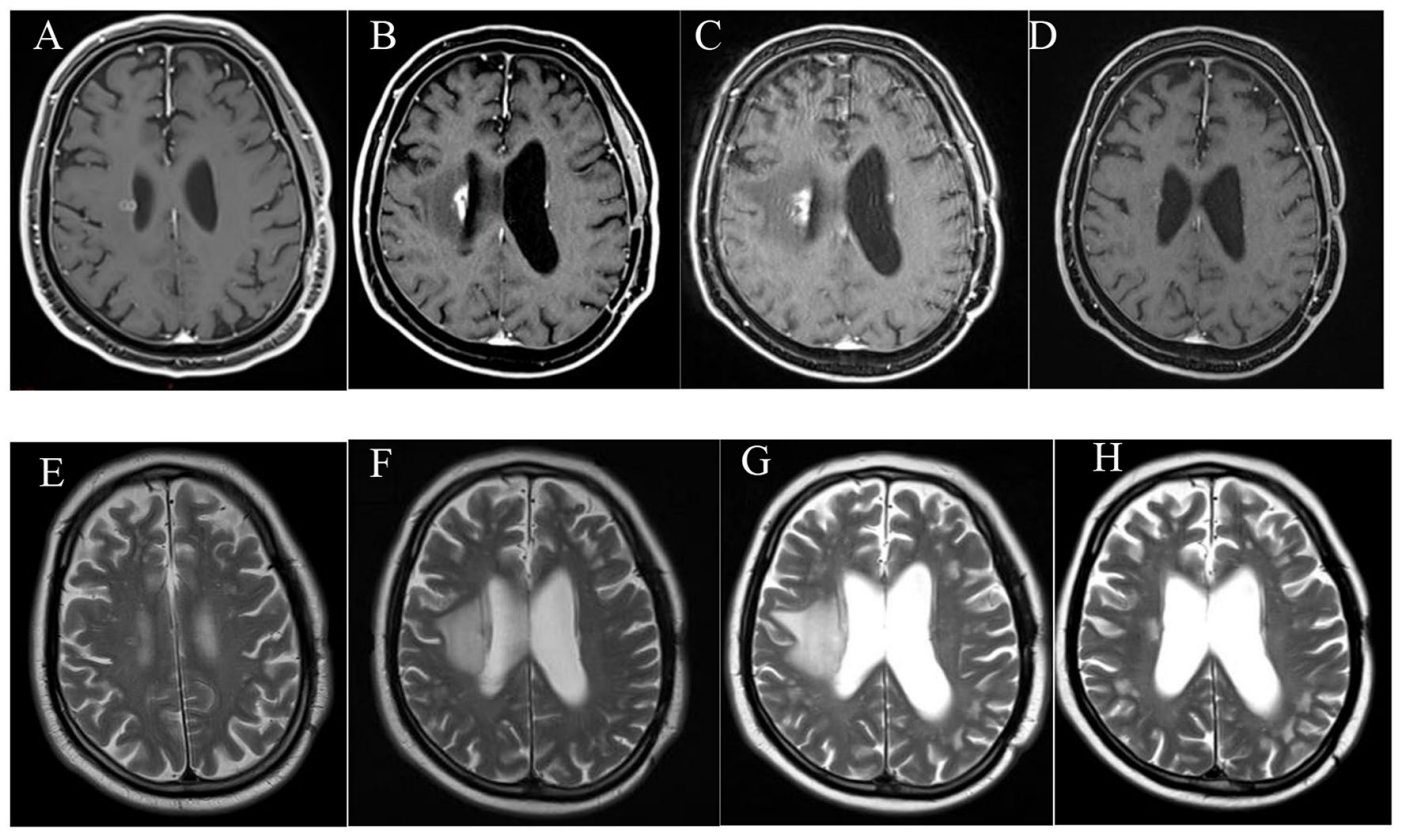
MRI images of a lung cancer patient with BM developing RN after SRS and treated with corticosteroids and BV. (A) Metastatic lesion adjacent to right lateral ventricle, (B) T1 weighted contrast enhanced lesion at the time of RN diagnosis. (C) T1 weighted contrast enhanced lesion after 1 month, after use of high dose dexamethasone. (D) T1 weighted contrast enhanced lesion after 3 months, after use of BV. (E) T2 weighted image showing metastatic lesion adjacent to right lateral ventricle. (F T2 weighted image showing edema at the time of RN diagnosis. (G) T2 weighted image showing edema after use of high dose dexamethasone. (H) T2 weighted image showing edema after use of BV
